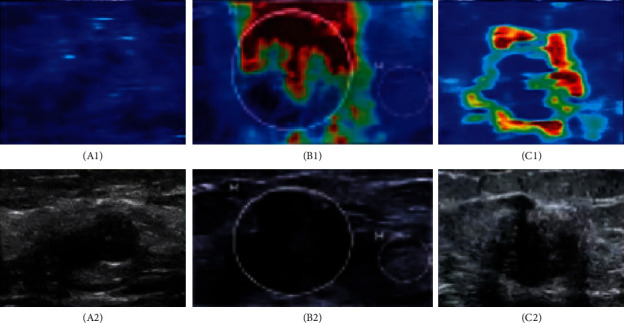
Comparison of ultrasound images of benign and malignant breast lumps. *Note.* A_1_ ∼ C_1_ were elastic ultrasound images; *A*_2_ ∼ C_2_ were B-mode ultrasound images; A_1_ and *A*_2_ show benign lumps; B_1_, B_2_, C_1_, and C_2_ show the malignant lumps.
